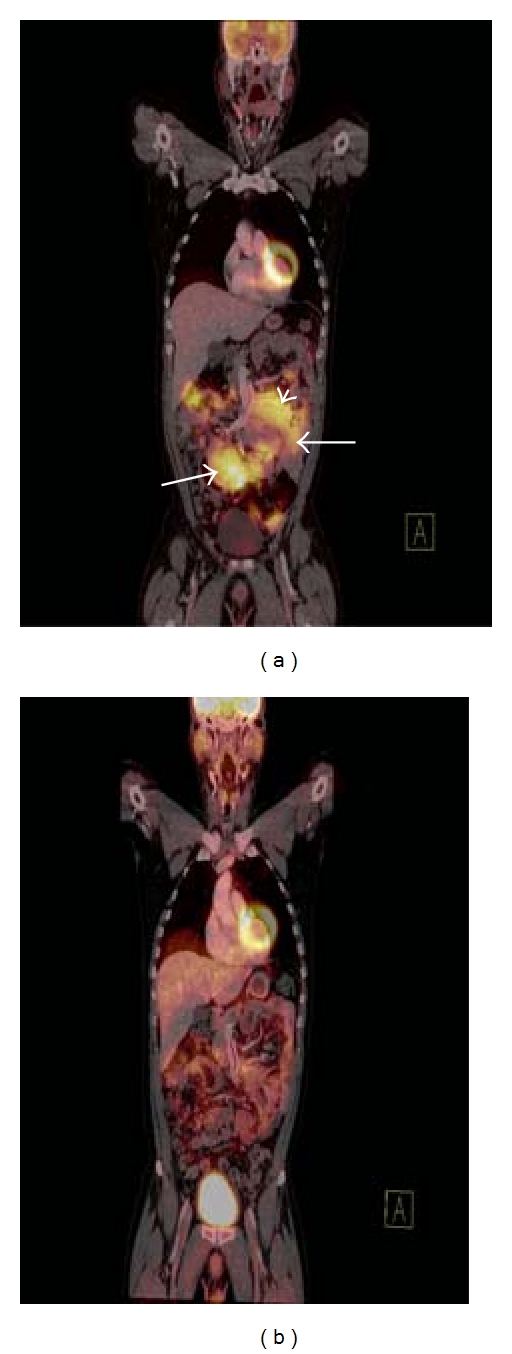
Coronal fused FDG-PET/CT image (a) demonstrating increased radiotracer uptake in multiple intra-abdominal lymph node masses in a patient with DLBCL (white arrows). Note how a large soft tissue mass displaces the small bowel and the mesenteric vessels (white arrowhead). Coronal fused FDG-PET/CT image (b) in the same patient demonstrating complete resolution of the previously described soft tissue masses. The observed residual FDG avidity is within bowel and is normal. This FDG-PET/CT confirms complete response to treatment.
